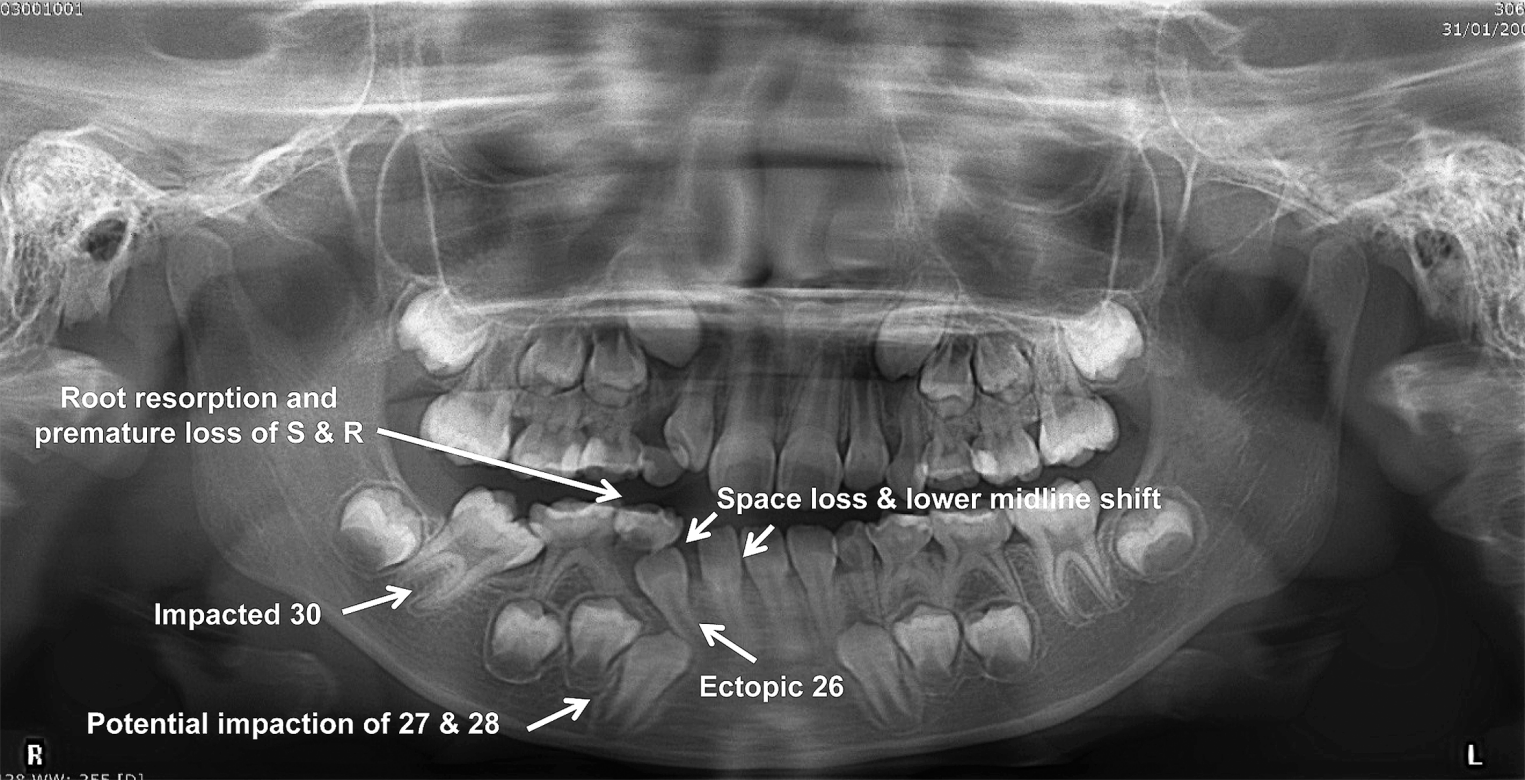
Panoramic radiograph of an 8-year-old boy showing the complications associated with the occurrence of an unerupted and ectopic #26.
